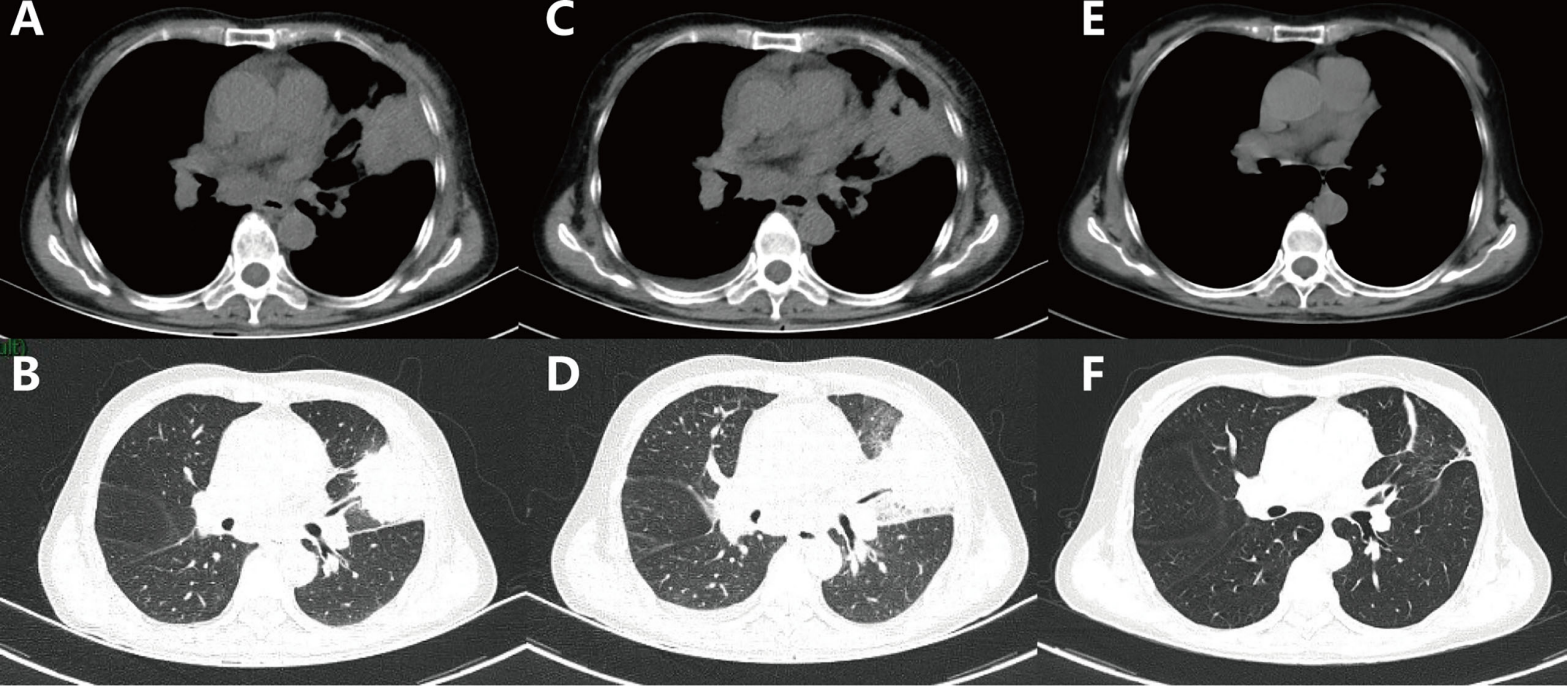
Computed tomography scans of the chest showed the following: (A, B) Baseline: lesion in the left lung before treatment. (C, D) One month after the use of osimertinib. (E, F) Thirteen months after the use of erlotinib combined with capmatinib.
